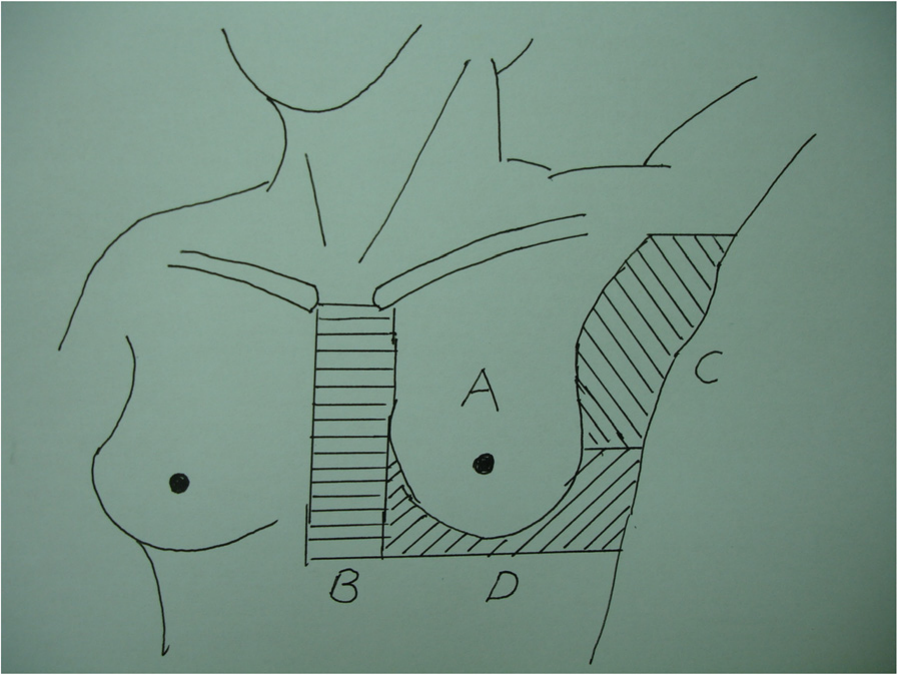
The 4 parts of irradiated area: A: main breast area; B: sternal/parasternal area; C: axillary area; D: inframammary area.
